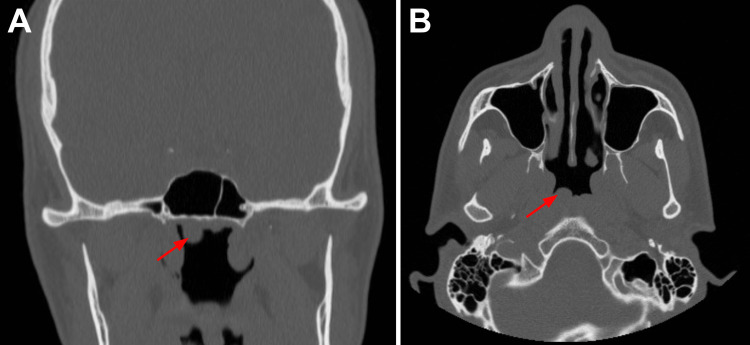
Postoperative CT scans of the Tornwaldt cyst Coronal (A) and transverse (B) views of CT scans showing a solitary Tornwaldt cyst (red arrow), 8 mm in diameter, along the posterior wall of the nasopharynx
